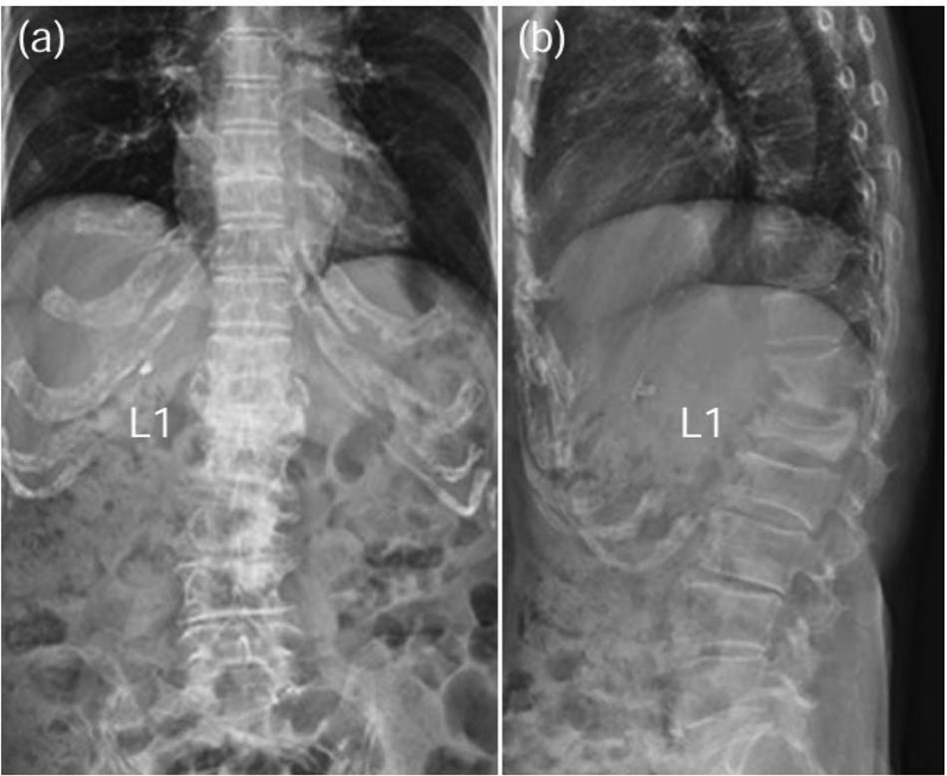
Plain radiographs obtained 6 months post-injury showed a cleft in the L1 vertebral body [anterior–posterior view: (a), lateral view: (b)].
